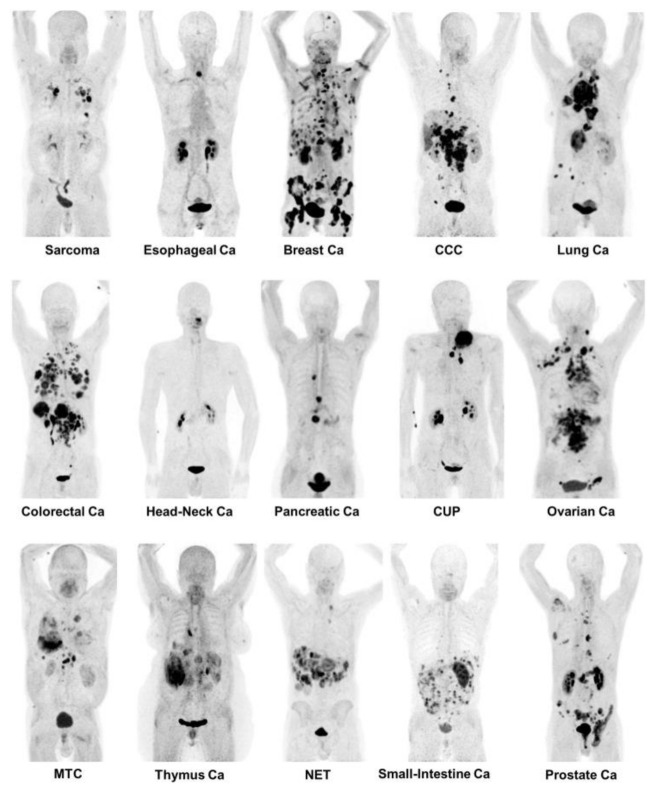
Depiction of Maximum Intensity Projections of several patients undergoing FAPI-PET/CT including a variety of different tumor entities. Adapted with permission from ref. [79].
